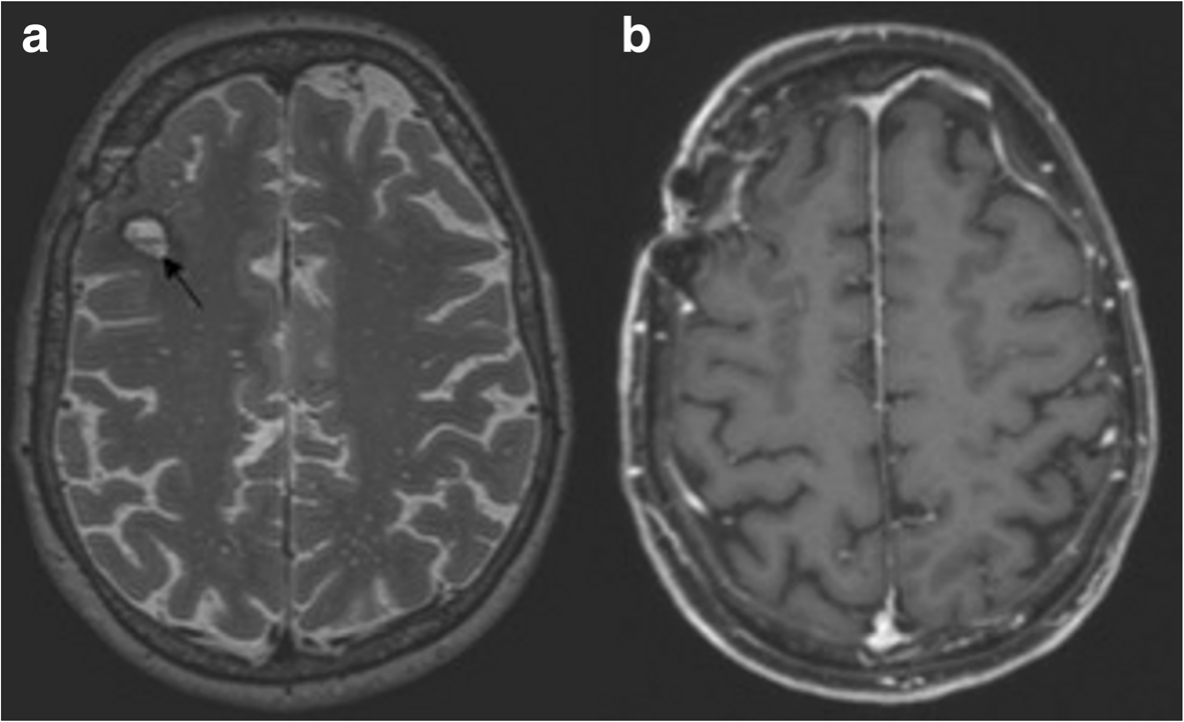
a and b Final magnetic resonance imaging (MRI) of the brain. Follow-up MRI performed 5 months after symptom onset demonstrated complete regression of the previously documented confluent white matter lesions on the axial T2-weighted (3 mm) image (a) and the contrast enhancement in the T1-weighted (3 mm) image after contrast application (b). At the site of the earlier biopsy, a small liquor isointense parenchymal defect with a T2-weighted hypointense hemosiderin rim and residual contrast enhancement of the biopsy cavity was seen in the right frontal lobe (a; *arrow*)
